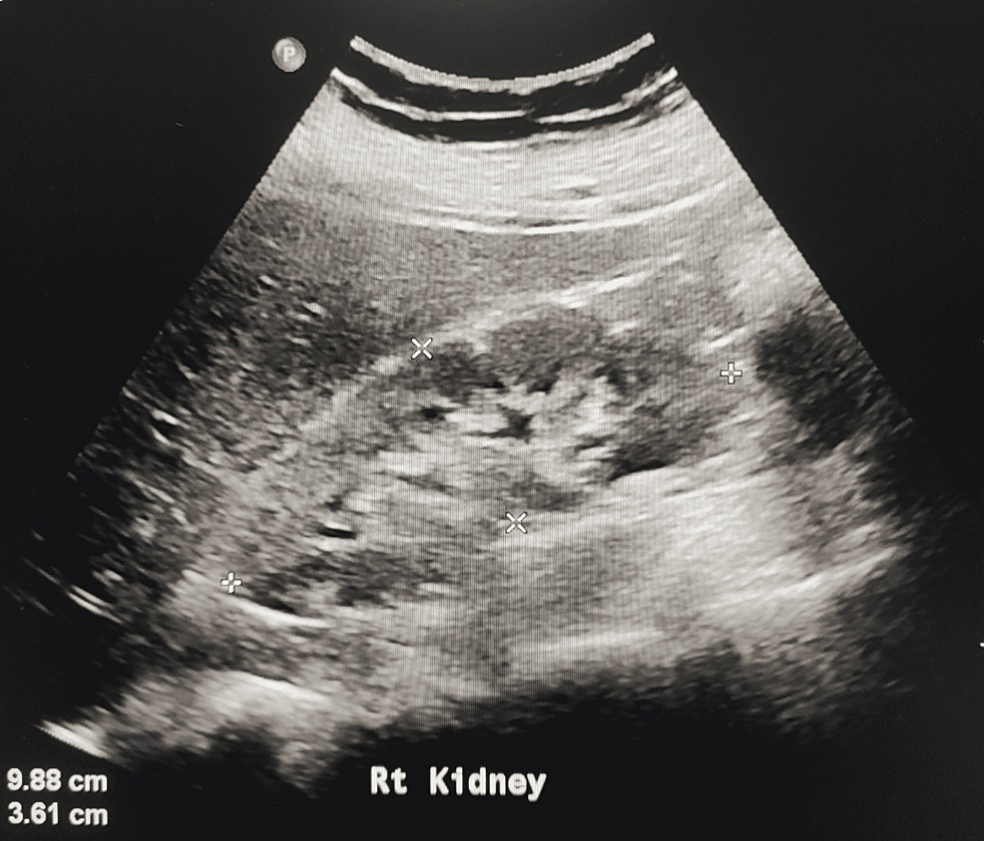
Ultrasound of the right kidney showing increased cortical echogenicity
